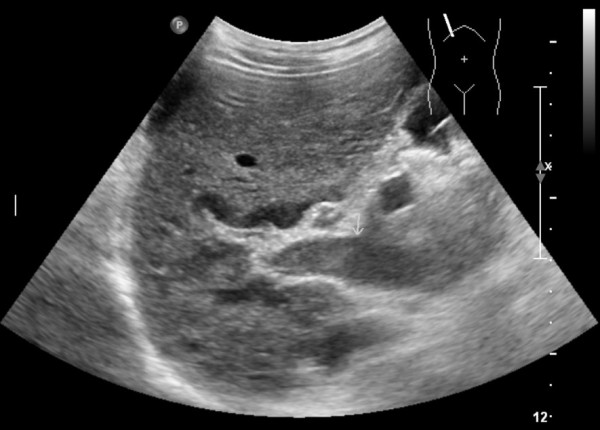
Abdominal ultrasound revealed thrombosis in proximal portion of the portal vein.
